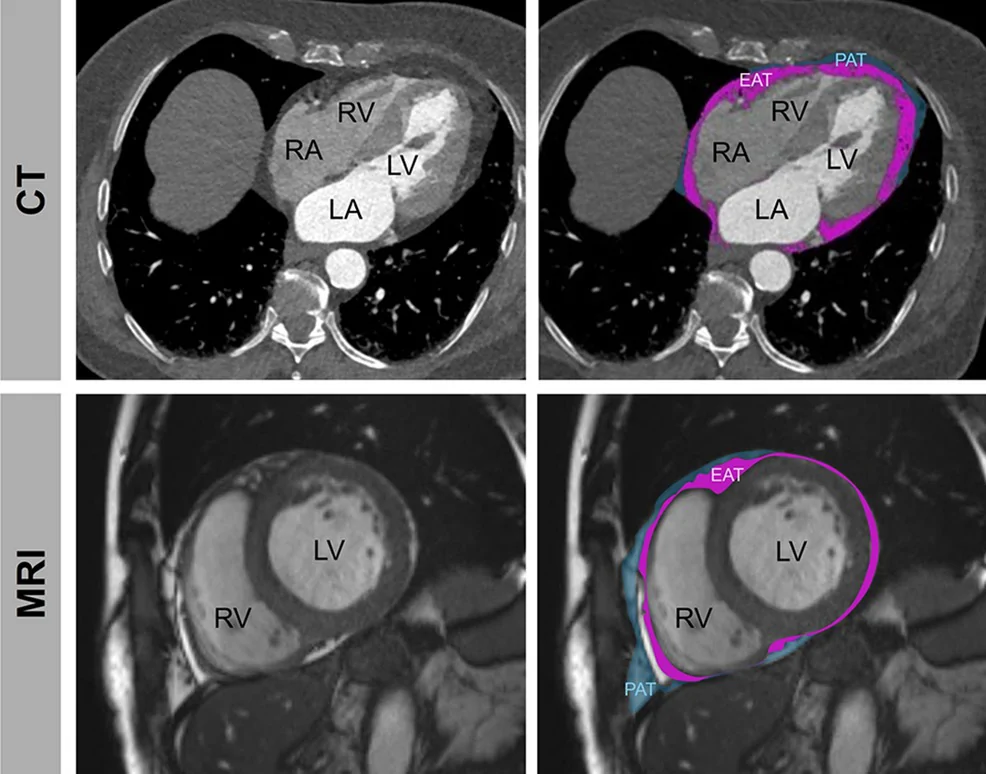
Representative visualization of epicardial adipose tissue (EAT) highlighted in pink and surrounding pericardial adipose tissue (PAT) highlighted in blue in both cardiac computed tomography (CT) and cardiac magnetic resonance imaging (MRI). LA, Left atrium; LV, Left ventricle; RA, Right atrium; RV, Right ventricle.
